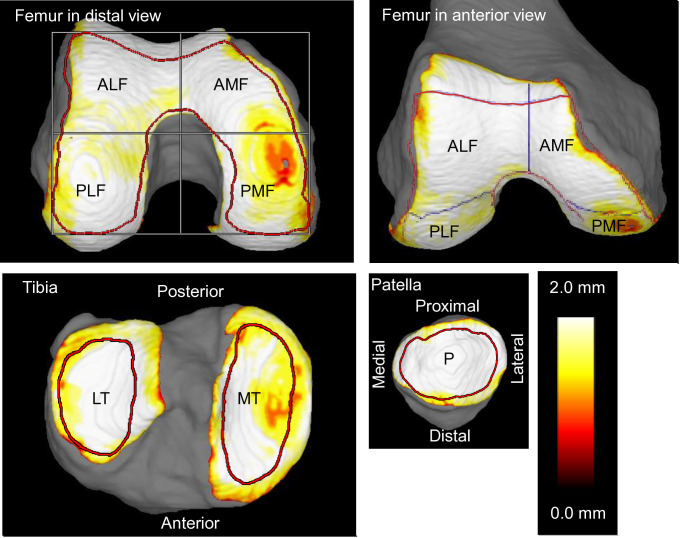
MRI cartilage thickness mapping and regions of the knee. The region of interest is indicated by a red line, and the femoral cartilage is divided into four regions by halving it vertically and horizontally in the distal view. PMF, posterior medial femoral; PLF, posterior lateral femoral; AMF, anterior medial femoral; ALF, anterior lateral femoral; MT, medial tibial; LT, lateral tibial; P, patellar.
